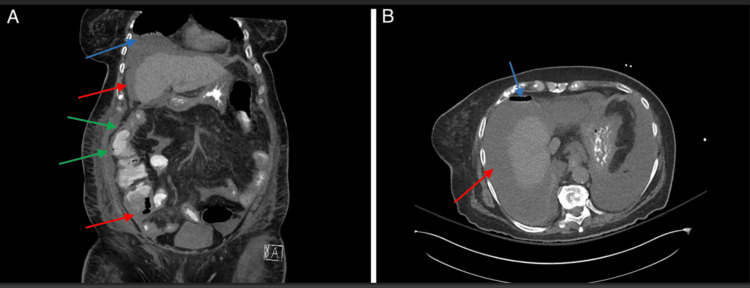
Representative images from CT abdomen/pelvis Pertinent findings include: (A) moderate volume of free fluid (red arrows), pneumoperitoneum (blue arrow), and pneumatosis (green arrows) of the ascending colon; and (B) free fluid (red arrow) and pneumoperitoneum (blue arrow). The presence of pneumatosis intestinalis and free air indicated colonic ischemia with perforation, corresponding clinically to the patient’s acute abdominal pain, hemodynamic instability, and need for emergent surgical intervention. These findings reflect late-stage complications of hypoperfusion and vasopressor-associated ischemia in the setting of multiorgan system failure.
